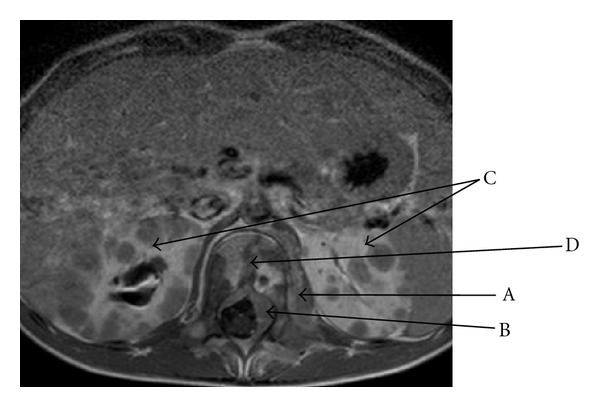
MRI (axial T1 gadolinium) lumbar paravertebral tumor (A) with epidural compression (B) through foraminae infiltration; bilateral nodular nephromegaly (C); heterogeneity captation of the vertebra (D), suggesting bone marrow infiltration.
